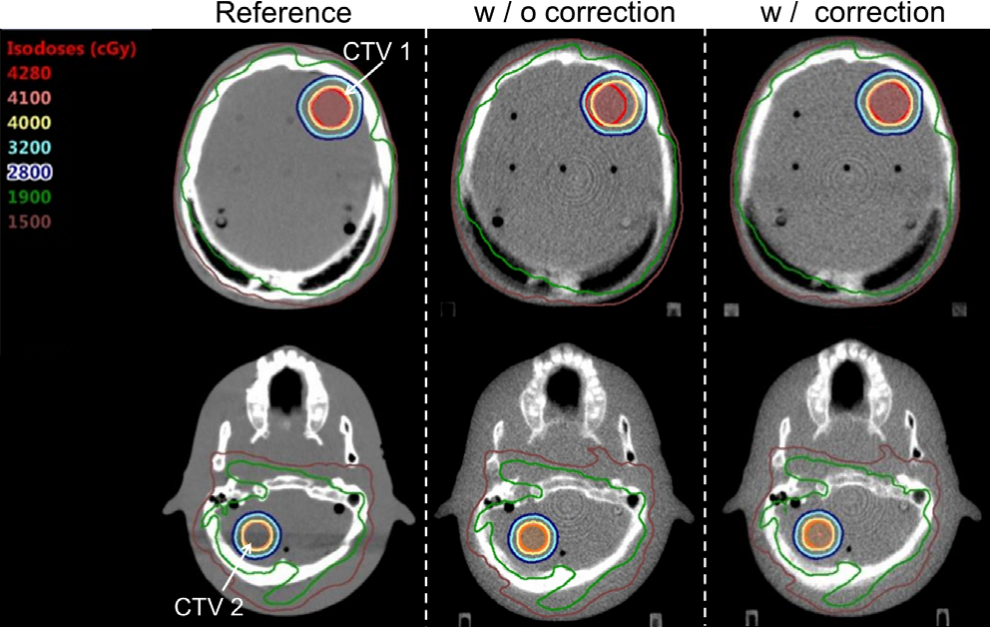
Dose distributions in whole brain + simultaneous integrated boost (SIB) stereotactic radiotherapy calculated on multi‐slice computed tomography (reference) and megavoltage computed tomography (with/without rotational correction).
